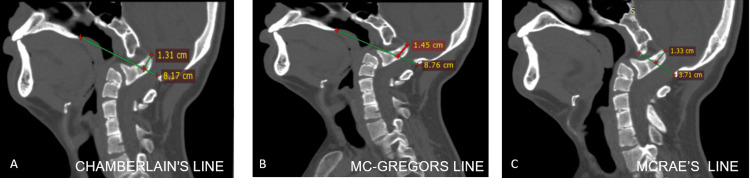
CT sagittal reformatted image demonstrated (A) the tip of the odontoid was 13.1 mm above Chamberlain's line, (B) the tip of the odontoid was 14.5mm above McGregor's line, and (C) the tip of the odontoid was positioned 13.3 mm above McRae's line. The above features suggested a diagnosis of basilar invagination.
